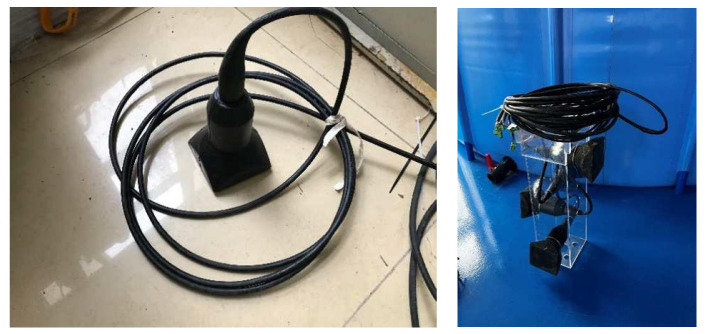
Proposed ultrasonic transducer.
